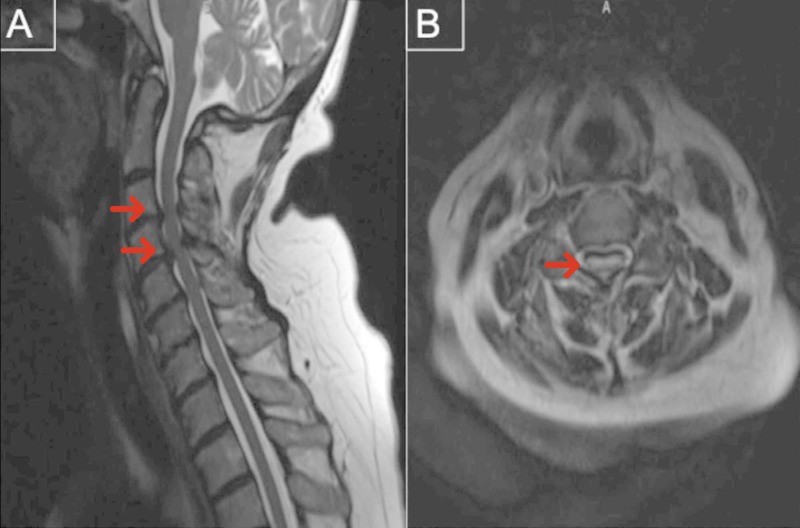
T2-weighted MRI of the sagittal (A) and axial (B) cervical spine revealing severe spinal cord compression at C4/5 with intramedullary spinal cord T2-signal.
